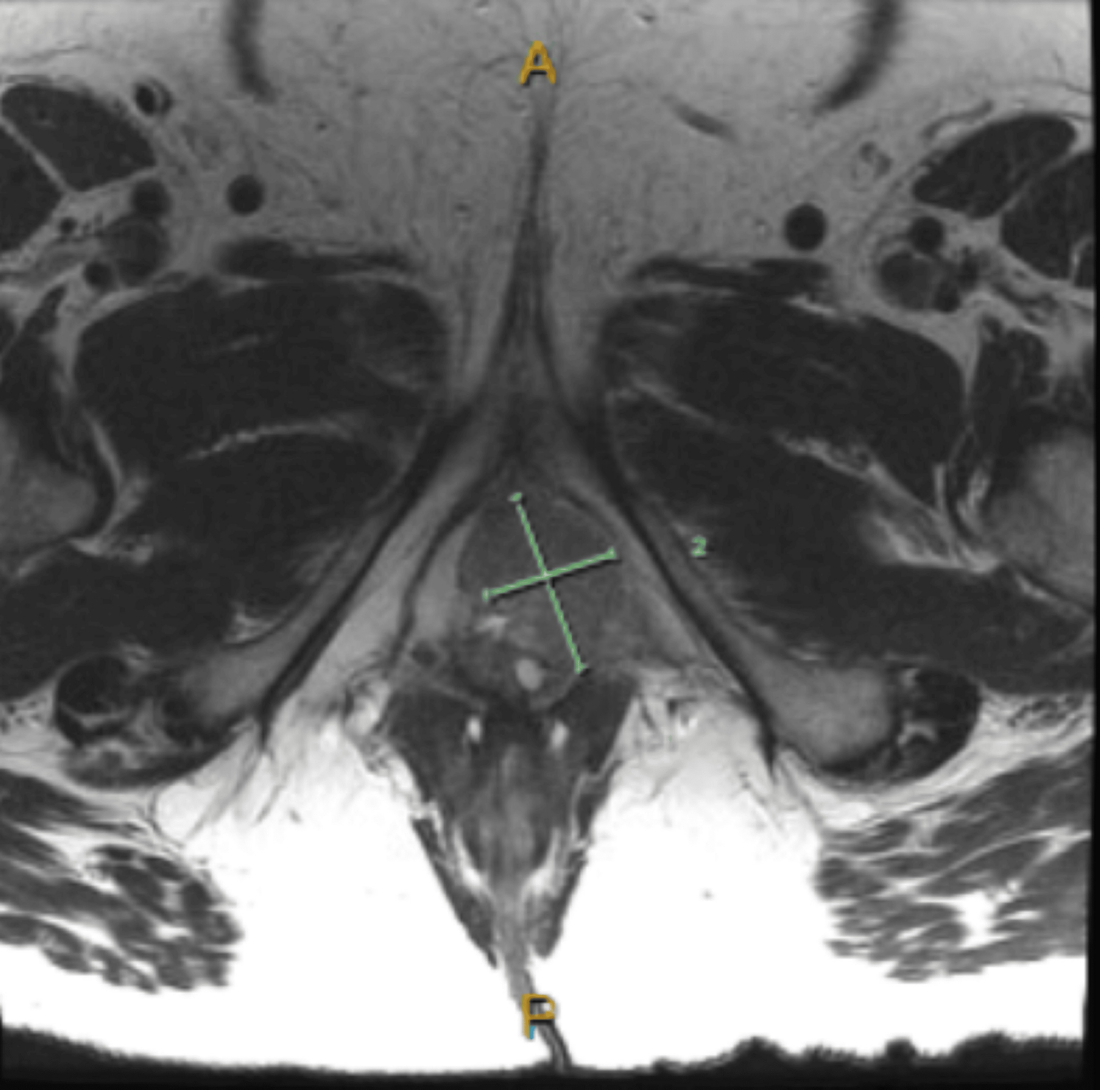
Transverse section of the pelvis MRI demonstrating the malignancy measuring 4.3 cm x 2.7 cm.
